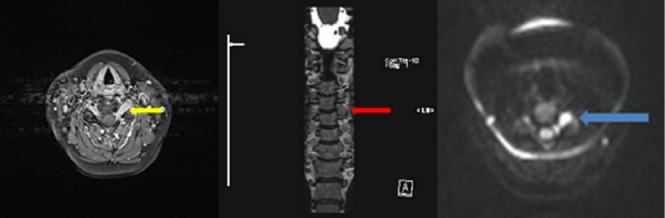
Initial MRI on presentation *Left to right* - *1st image: *Axial T1-weighted fat-sat post-contrast image shows asymmetric enlargement and uniform enhancement of the left C4-5 nerve root (yellow arrow).* 2nd image: *Corresponding coronal T2-weighted image showing the lesional enhancement (red arrow). *3rd image - *The nerve root shows intense diffusion restriction of diffusion weighted imaging (blue arrow).
